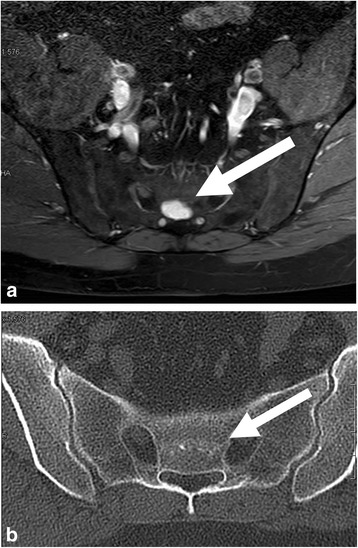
a-c 41-year old patient with solitary metastatic disease in the sacrum (S3) 6 years after surgery of the primary in the right calf. Lesion clearly detectable on MRI (a) but not on CT (b) or PET-CT scan (c)
